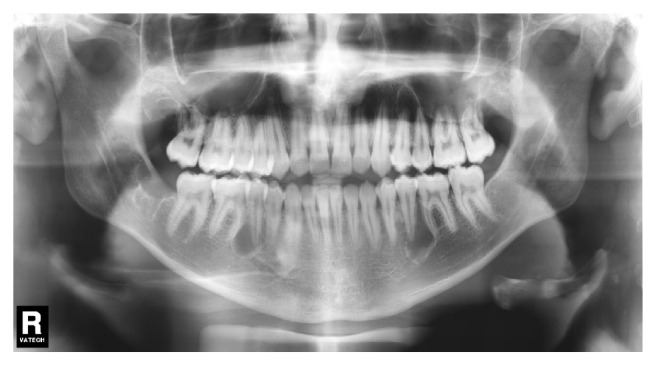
Panoramic radiograph of the patient aged 18 years after the extraction of all supernumerary teeth and third molars.
